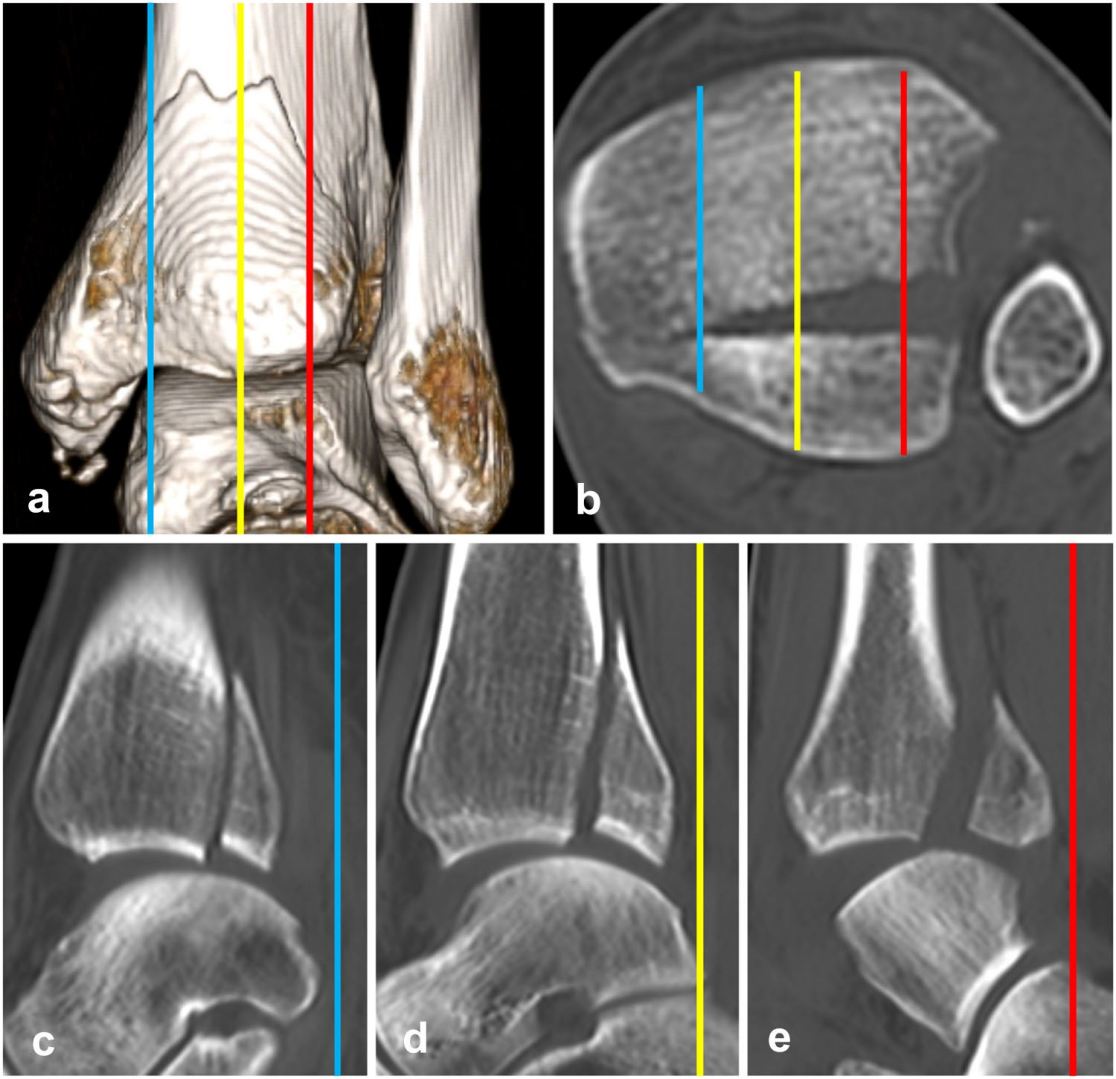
The size of PM fragment and its articular surface depending on location sagittal CT scans. a 3D CT posterior view; b axial CT scans; c medial (blue line) sagittal CT scan; d central (yellow line) sagittal scan; e lateral (red line) sagittal scan
